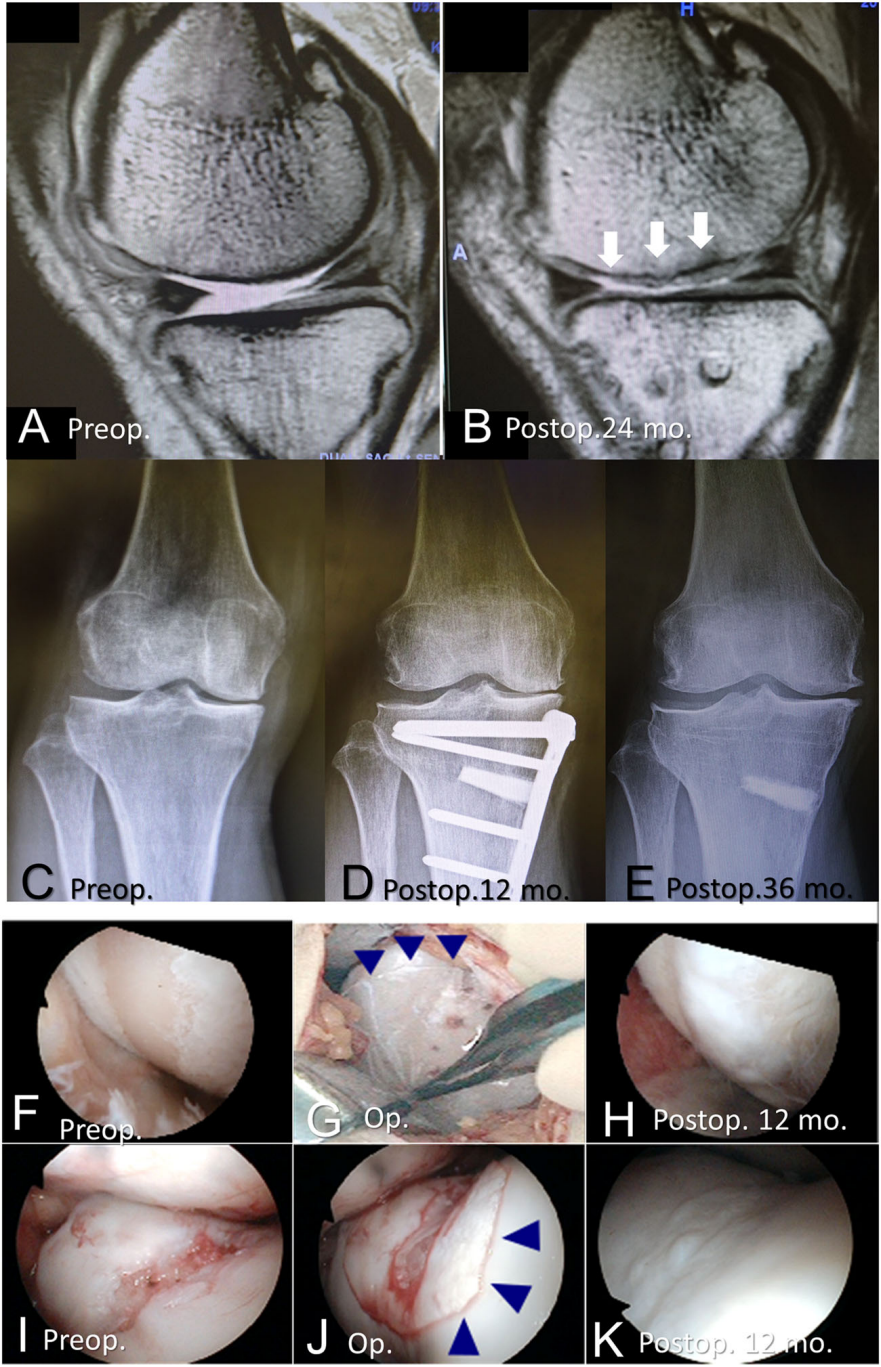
Representative magnetic resonance imaging (a, b), X-ray photographs (c–e), and arthroscopic images (f–k). a–e (patient 8), f–h (patient 6), and i–k (patient 2). a, b T2-weighted images were obtained using a 3.0-Tesla instrument. a Lack of articular cartilage and accumulation of synovial fluid were observed preoperatively. b Regeneration of articular cartilage, indicated by the arrows, was confirmed 24 months postoperatively. c X-ray photographs confirmed a preoperative varus deformity and narrowing of the medial joint space. d X-ray photographs 12 months postoperatively showed that the alignment was maintained after OWHTO. e At 36 months postoperatively (24 months after implants were removed), the alignment was maintained and the β-tricalcium phosphate graft had been absorbed. The medial osteophyte progression was observed in this patient. f–h The cartilage defect of the medial femoral condyle caused exposure of the subchondral bone. i–k The cartilage defect in the trochlear groove of the femur is shown. f, i Preoperative cartilage defects are shown. g, j Chondrocyte sheets were transplanted. h, k The presence of regenerated cartilage was confirmed at 12 months postoperatively
